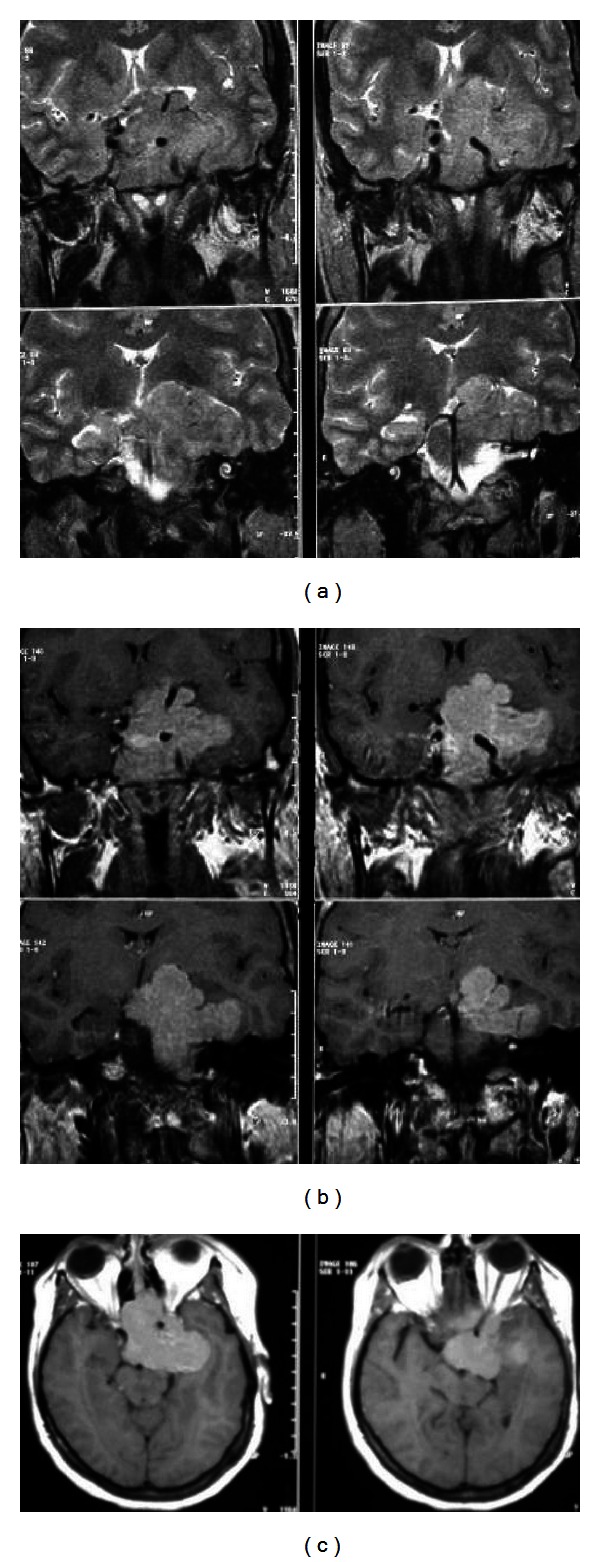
(a) Coronal T2-weighted turbo spin echo magnetic resonance image shows solid macroadenoma filling the entire sella, invading left cavernous sinus, and obliterating the suprasellar cistern; (b)-(c) postcontrast coronal (b) and axial (c) spin echo images show that the mass enhances intensely and homogenously. The extention of the macroadenoma in the prepontine cistern and the encasement of cavernous portion of the left internal carotid artery were more prominent in these images.
